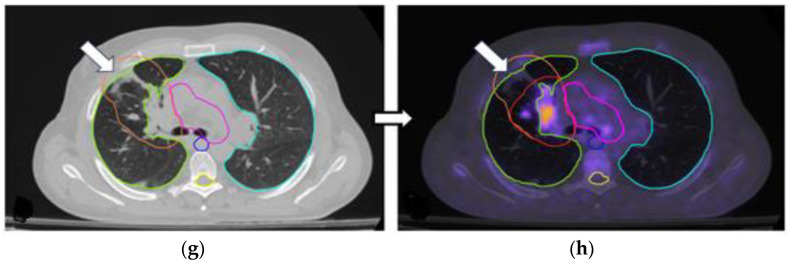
Representative CT (left column) and PET-CT (right column) images showing alterations in planning target volume (PTV) based on PET-CT findings. White arrows highlight PTV alterations. (a,b) Expansion of the tumor PTV (orange) in PET-CT to include an adjacent metabolically active region (red) not clearly seen on CT. (c,d) Addition of nodal PTV (orange) in PET-CT to include a previously undetected subcarinal lymph node. (e,f) PET-CT-guided expansion of the tumor PTV to cover a pleural lesion missed by CT imaging. (g,h) Reduction in the PTV in PET-CT to spare normal lung tissue previously misclassified as a tumor due to atelectasis. Green and white blue margin shows left and right lungs, corresponding; yellow margin shows spinal cord; blue margin shows trachea and pink margin shows heart based CT (left column) and PET-CT (right column).
